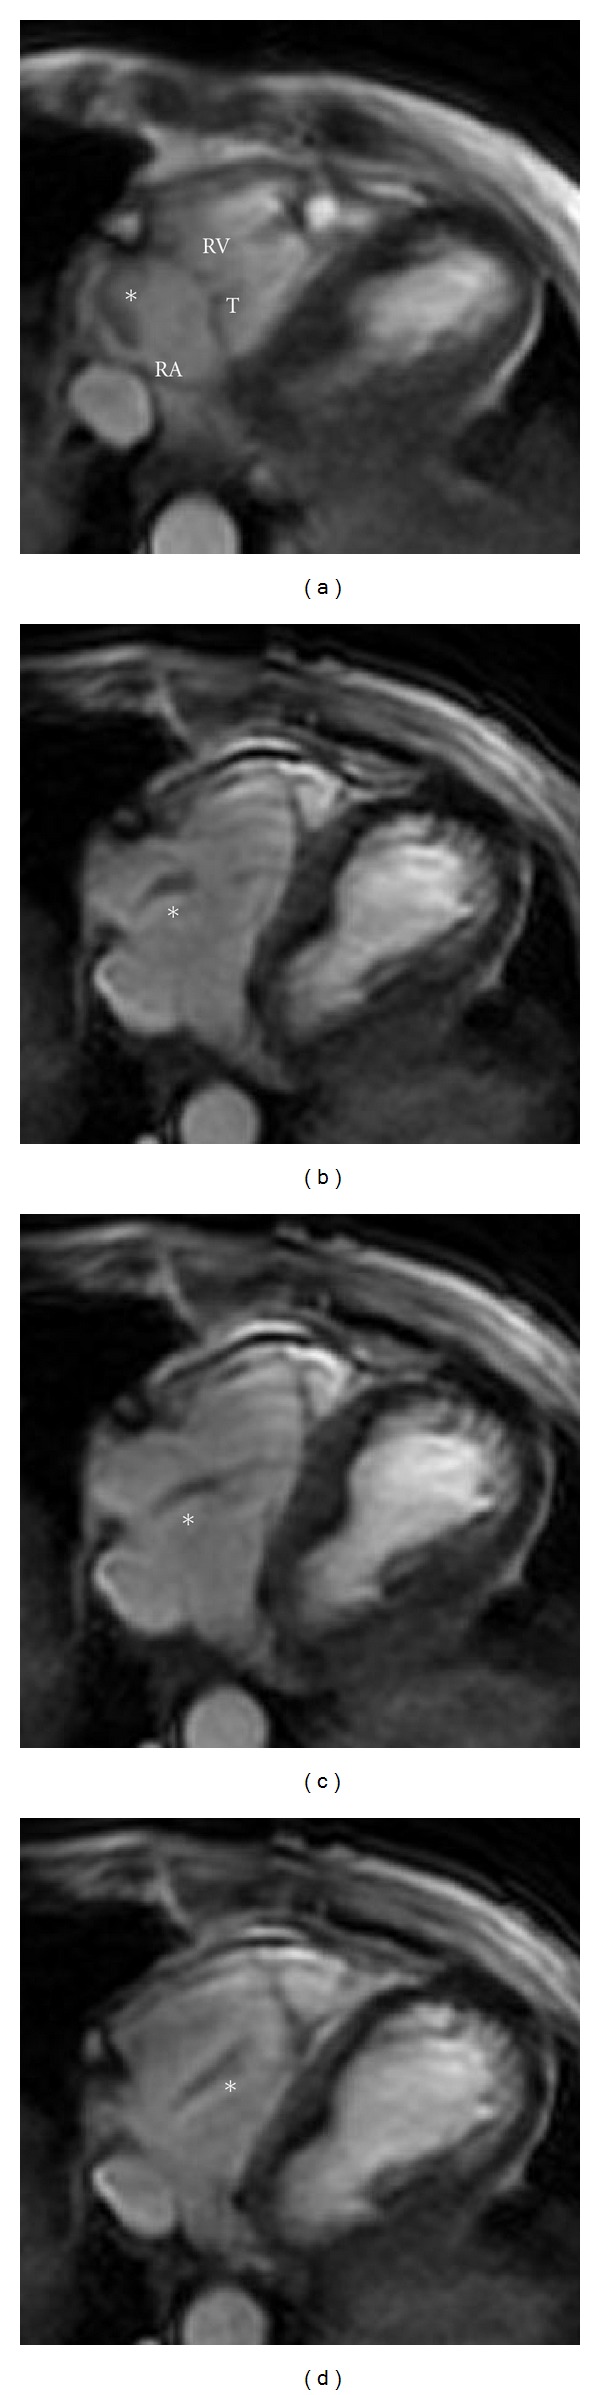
MRI. Sequence of the movement of the thrombus (*) in the right heart. During the systole is “rolled” in RA (a), in isovolumic diastole “lengthens” ((b), (c)) to then prolapse in RV, in end-diastole (d).
